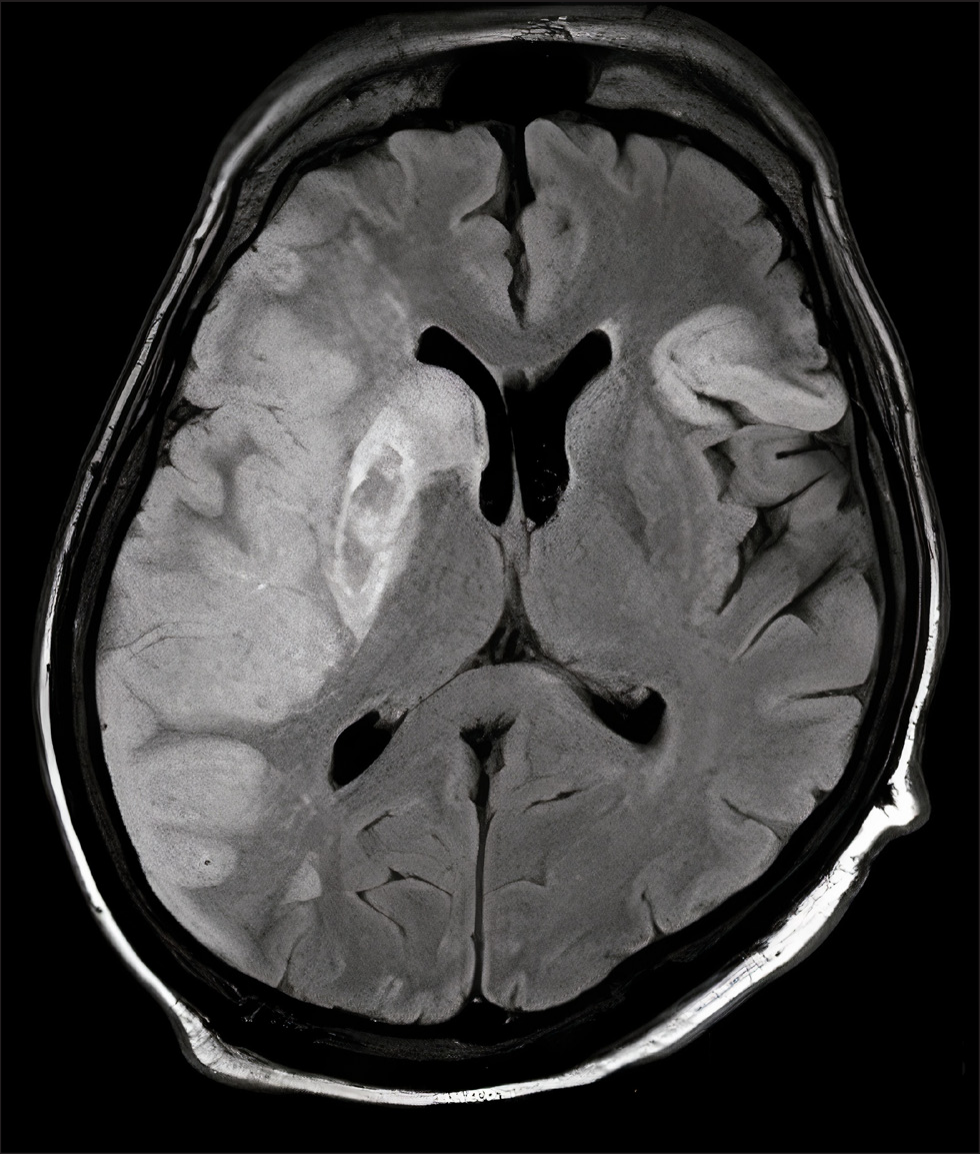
Magnetic resonance imaging of head indicating right-sided middle cerebral artery stroke.
